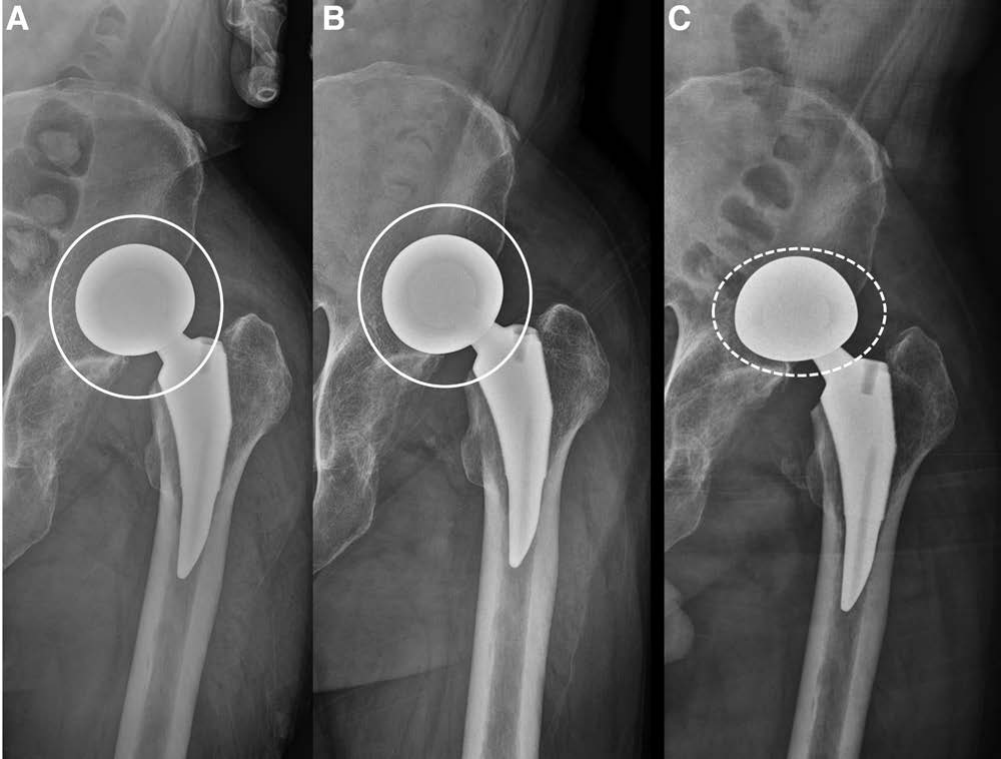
Anteroposterior radiograph demonstrating dislocation in hip hemiarthroplasty. (A) The first dislocation occurred 8 wk following surgery, and the femoral head component appeared as an “O”-shaped concentric circle. (B) The second dislocation occurred 2 d after the first dislocation. The femoral head component appeared as an “O”-shaped concentric circle. (C) The third dislocation occurred. The femoral head component appeared elliptical.
